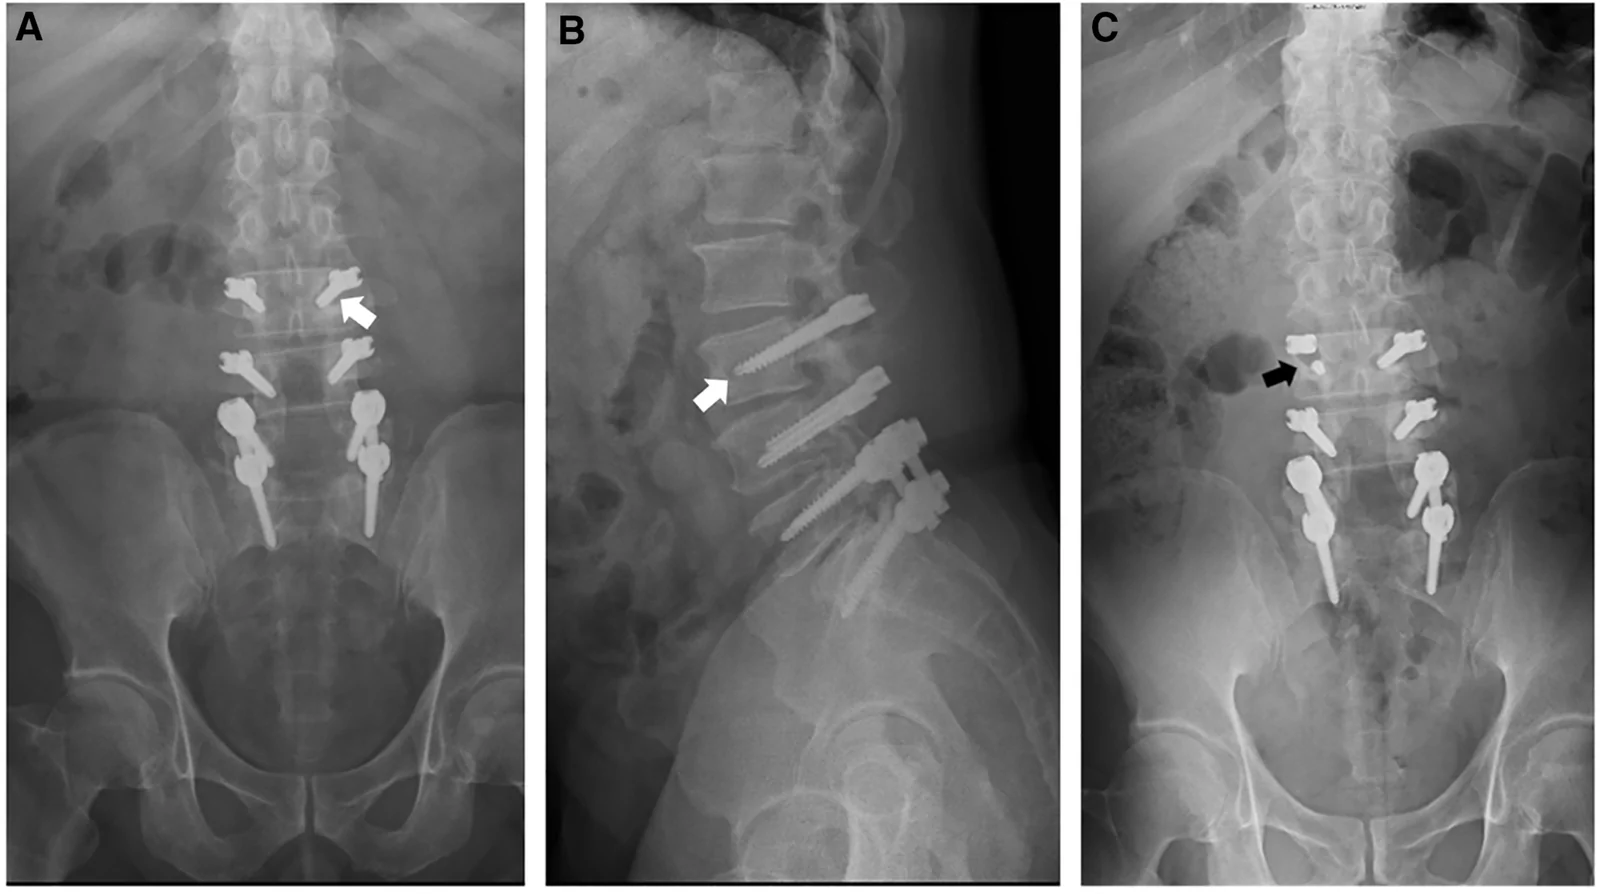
Radiographic findings in patients with late-onset infection after Dynesys Dynamic Stabilization. (A) Anteroposterior and (B) lateral plain radiographs showing pedicle screw loosening (white arrow) and mechanical instability of the dynamic stabilization construct. (C) Follow-up anteroposterior radiograph demonstrating a progressive screw fracture (black arrow), raising suspicion for deep peri-prosthetic infection.
